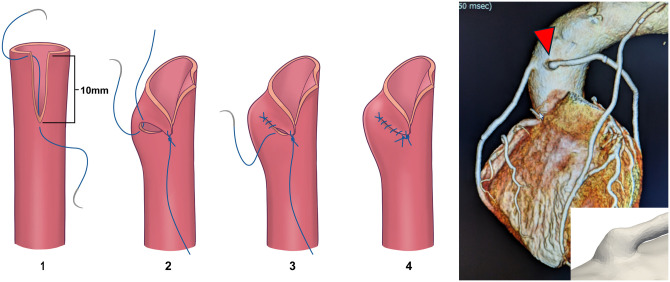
Graft Configuration Procedure and Images of Pouch Technique. (Left) The proximal stump of the internal thoracic artery was incised longitudinally for 10 mm. One edge of the incision was sutured to the opposite end using 7-0 polypropylene (1 and 2), and the folded sideline was closed with a continuous running suture (3 and 4). (Right) 3-Dementional CT scan image of free RIMA graft with magnified image of the proximal anastomosis using Pouch technique
